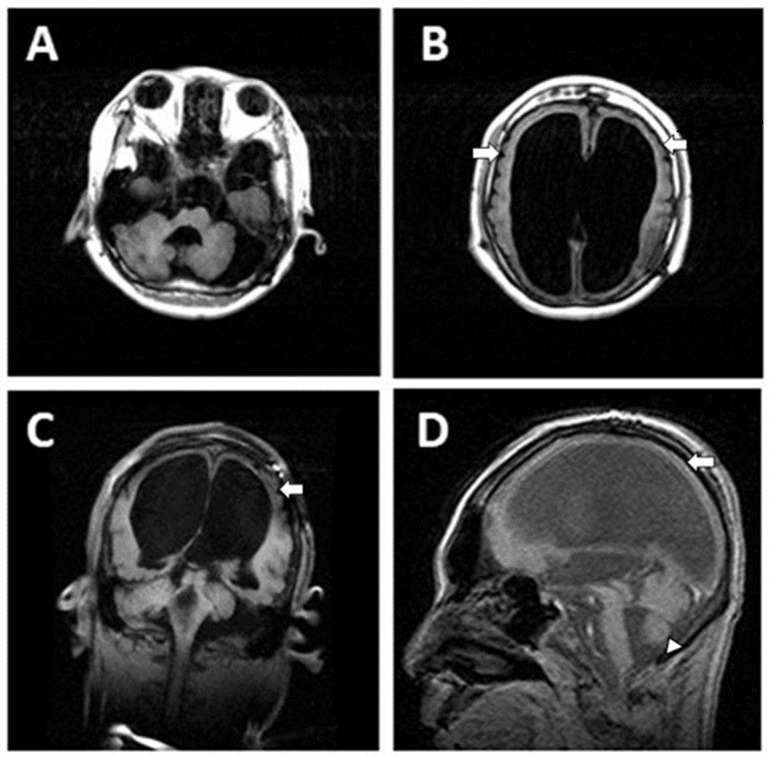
Brain MRI (T1WI) of Case 1 showed marked dilated ventricles with displaced cerebellum (A), dysgenesis of corpus callo-sum, pachy/polymicrogyria (white arrows in (B–D)) and small vermis (arrowhead in (D)).
